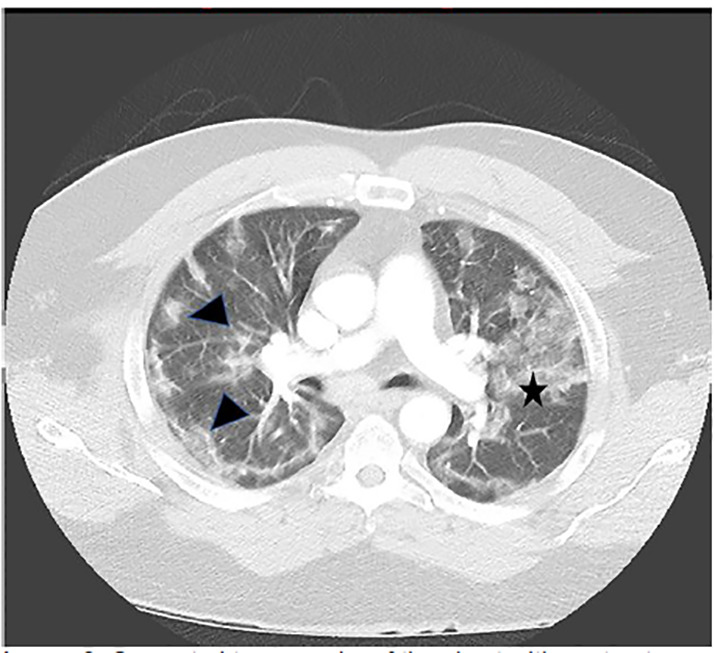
Computed tomography of the chest with contrast that demonstrates peripheral, ground-glass opacities (GGO) (arrowheads) in the periphery and a large area of GGO (star).
